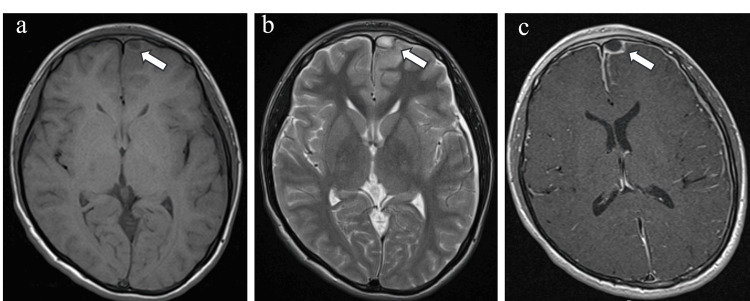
Axial Head MRI a) Horizontal MRI section showing a low signal on T1-weighted images, b) high signal on T2-weighted images in the left frontal epidural space with an approximate dimension of 10×8 mm, c) a uniform low-signal area with contrast effect in the surrounding dura mater on T1-weighted images with gadolinium contrast. A contrast effect was also observed in the frontal bone marrow.
